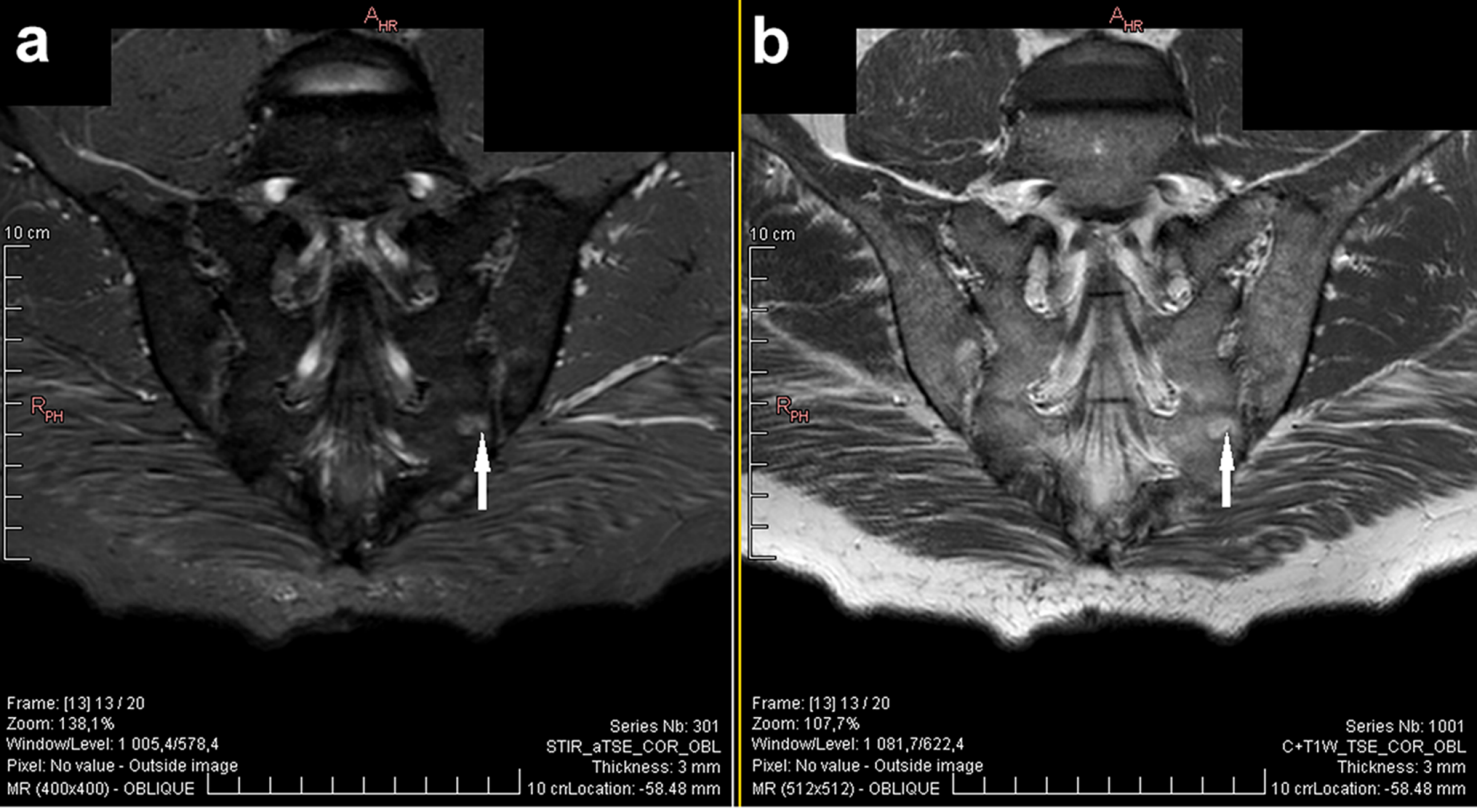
Typical axSpA bone-marrow oedema lesion (white arrow) in the lower sacral quadrant of the left sacroiliac joint on STIR sequence (a) and contrast-enhanced T1-weighted sequence (b). STIR, short tau inversion recovery
